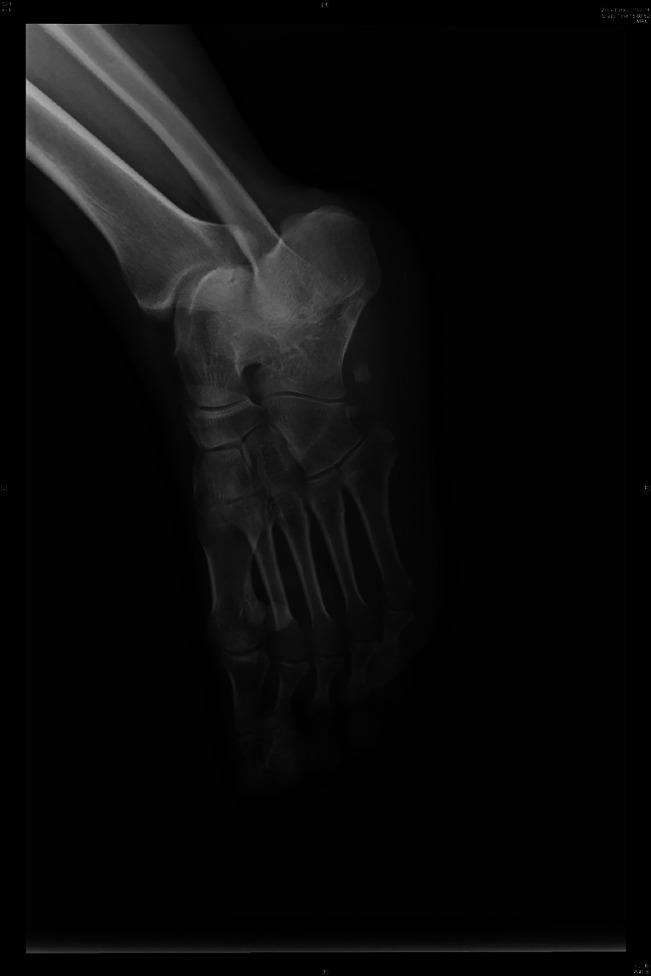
Preoperative radiograph showing a fifth metatarsal base fracture and displaced os peroneum fracture.
